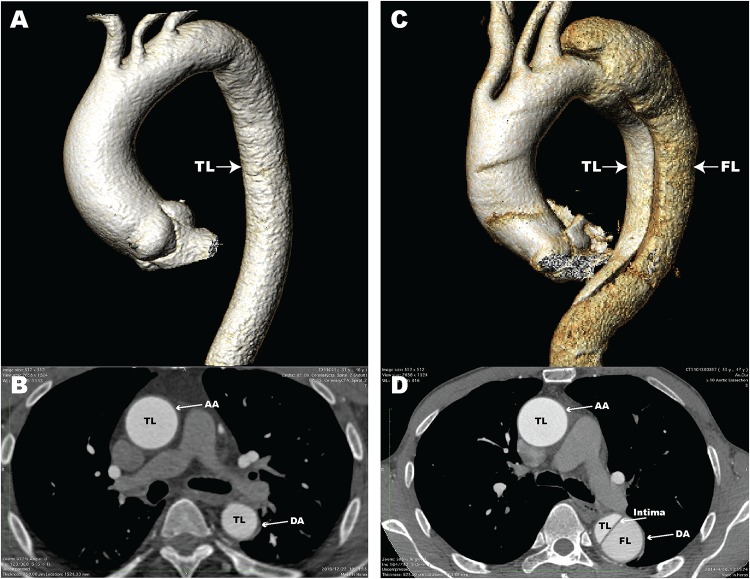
Computed tomography angiography (CTA) images of a normal aorta and an aortic dissection (AD). (A) 3D reconstructed CTA image of a normal aorta; (B) Axial CTA image of a normal aorta with blood flow only in the true lumen (TL); (C) 3D reconstructed CTA image from a patient with AD; (D) Axial CTA image of AD with blood flow in both the true lumen and false lumen (FL). The aortic intima is located between the TL and FL. AA, ascending aorta; DA, descending aorta.
